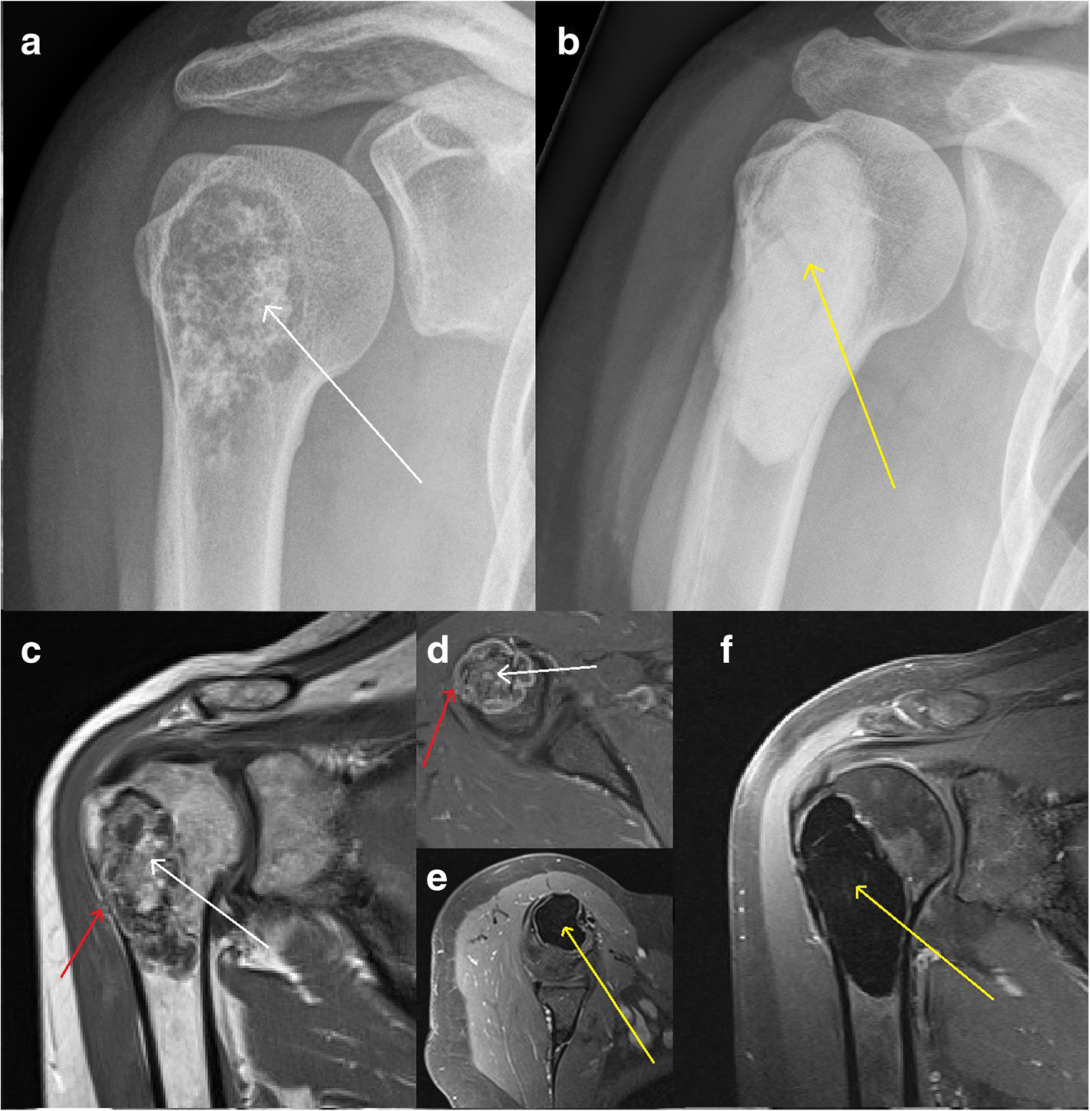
Surgical treatment at the proximal humerus Typical chondroid matrix (white arrows) is visible in ap x-ray (a) and coronal (c) and axial (d) T1 dotarem contrast-enhanced MRI. The decision to perform surgery was made due to local pain, lesion size and radiological signs of aggressiveness with endosteal scalloping (red arrows). After rigorous intralesional curettage and use of a high-speed burr, the lesion was filled with polymethylmethacrylate bone-cement (yellow arrows in b, e, and f).
